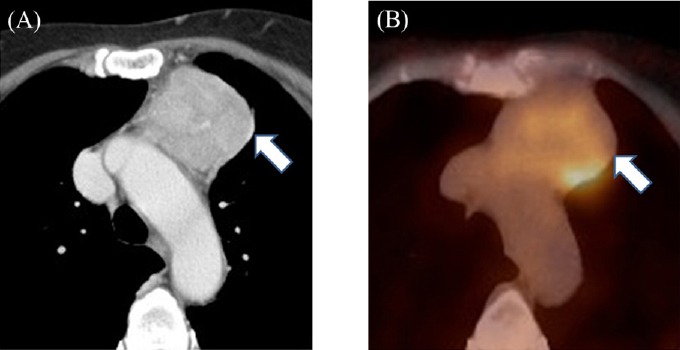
A 64-year-old woman with thymic carcinoma (A) Contrast-enhanced CT image shows a well-defined, homogeneous anterior mediastinal tumor (arrow); (B) PET/CT image shows high FDG uptake (arrow); SUV_max_, MTV and TLG estimated at 5.4, 27.7 mL and 88.7 g, respectively
